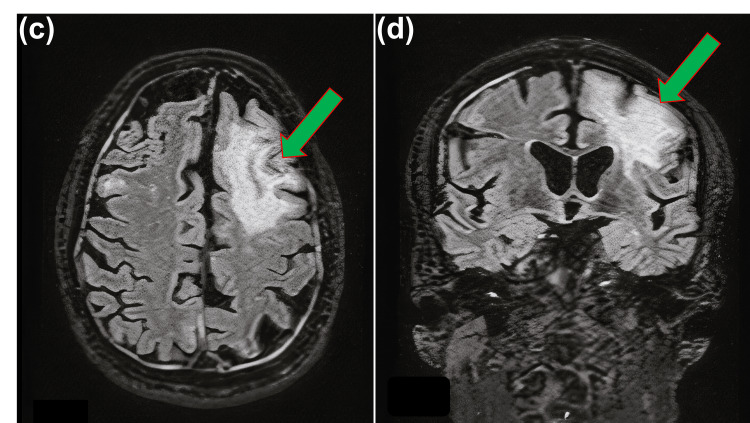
MRI images (c) axial flair, and (d) coronal flair. Axial and coronal flair showing the left frontal lobe lesion as observed in Figure 1.
